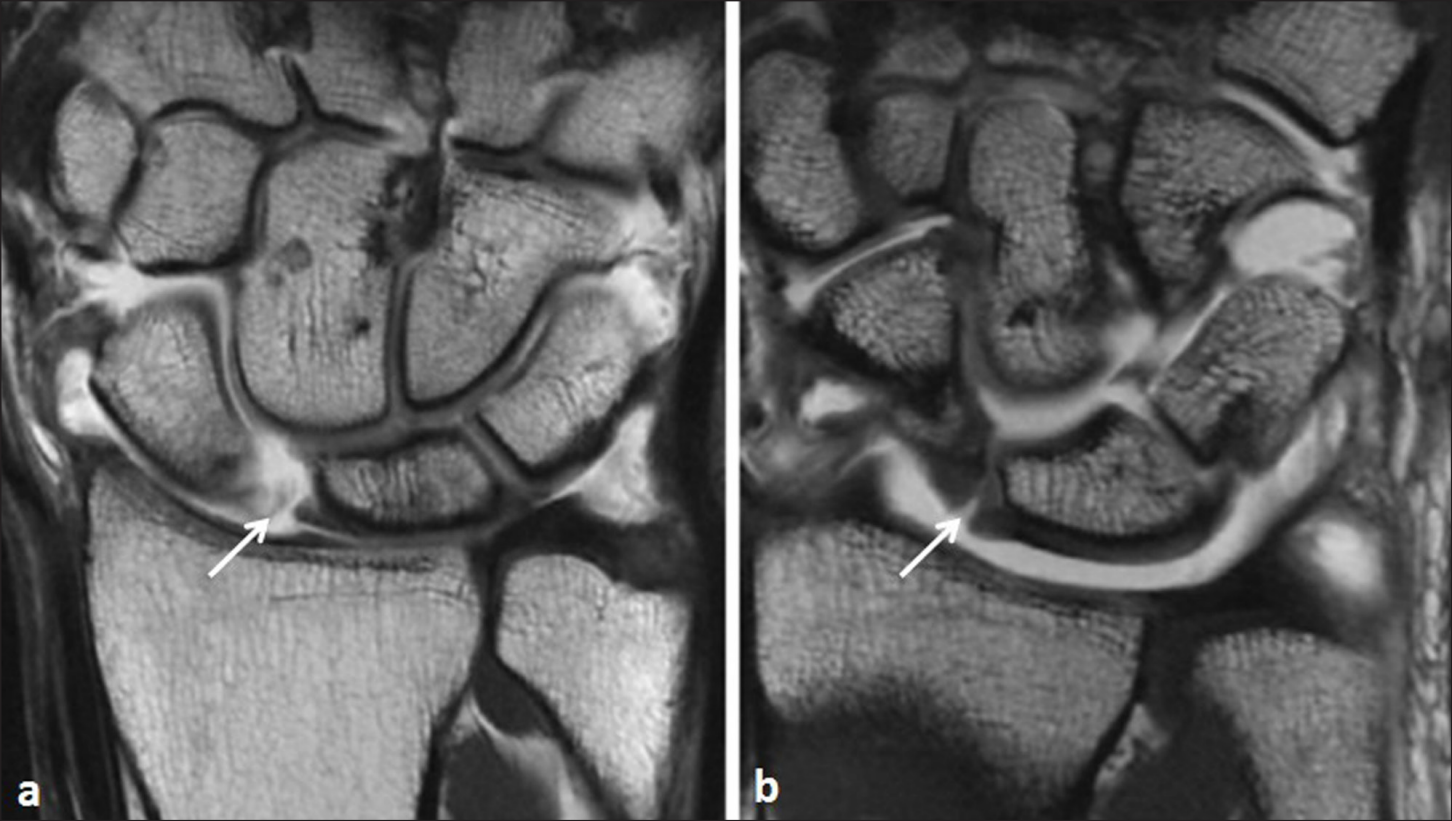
T1-weighted coronal images in two symptomatic patients with a scapholunate ligament tear. a. Without traction. There is marked widening of the scapholunar interval (*arrow*). b. With traction. Apart from the tear (*arrow*), there seems to be a step-off deformity in Gilula’s most proximal arc.
